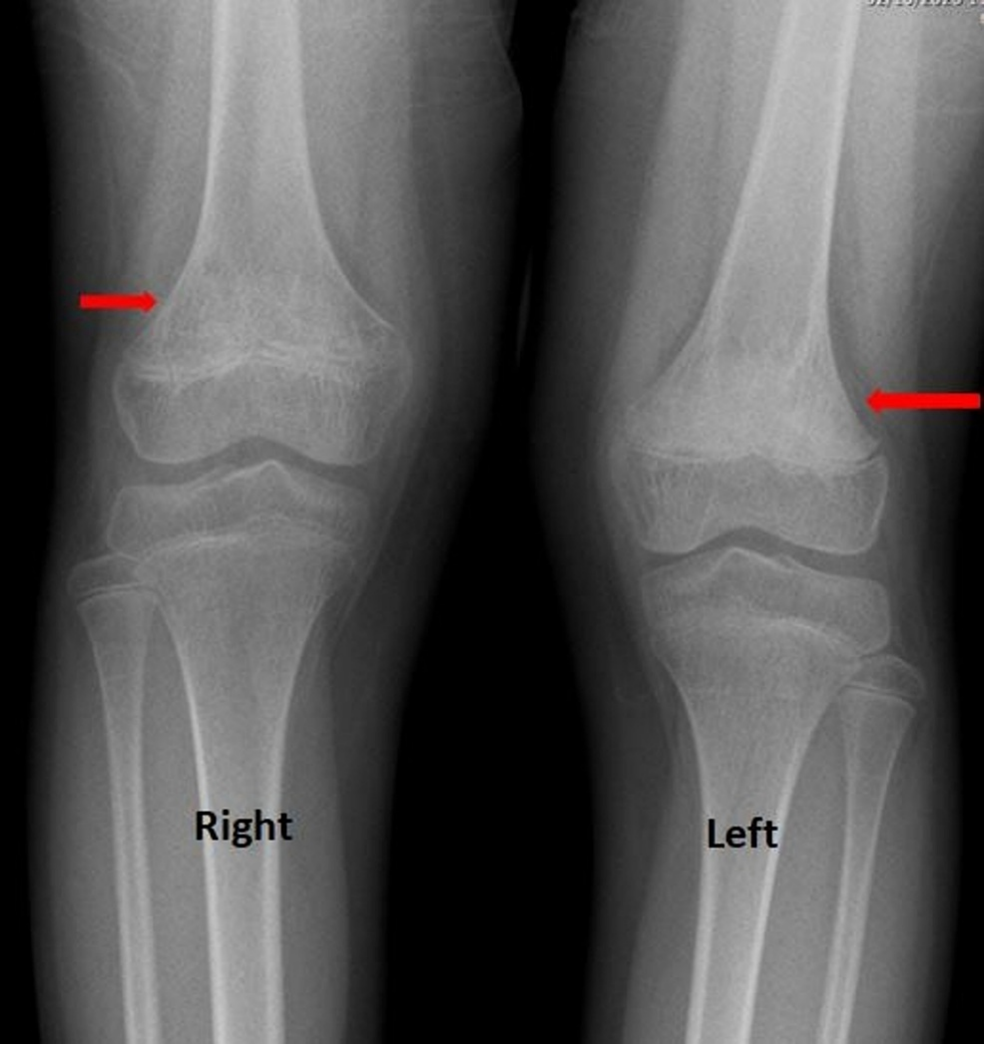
X-ray of both knees. It shows sclerosis in the distal femoral metaphysis, more severe on the left side (long red arrow) as compared to the right (short red arrow).
